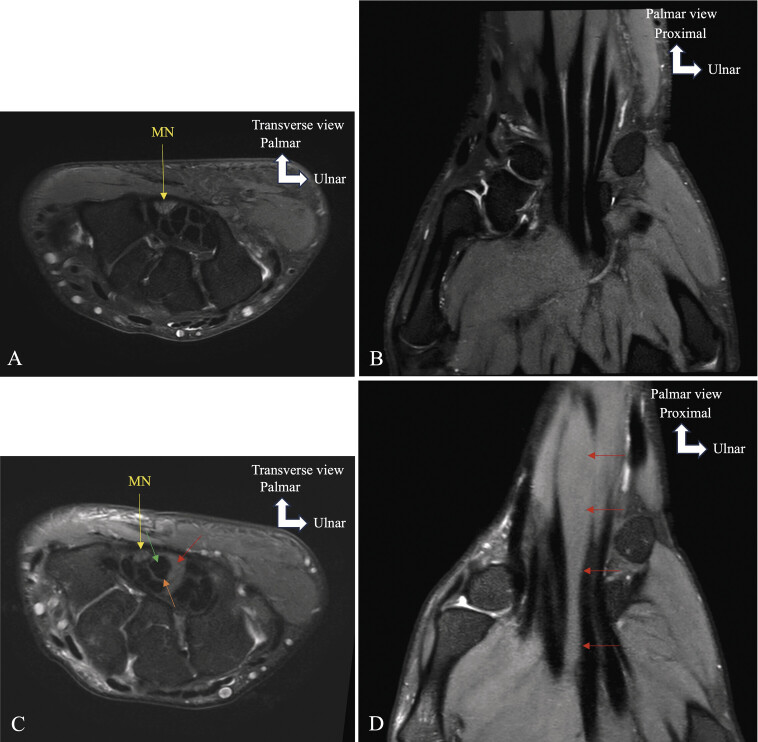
Distal extension of an accessory flexor digitorum superficialis muscle of the index finger into the carpal tunnel increases pressure, resulting in carpal tunnel syndrome. Axial MRI (
